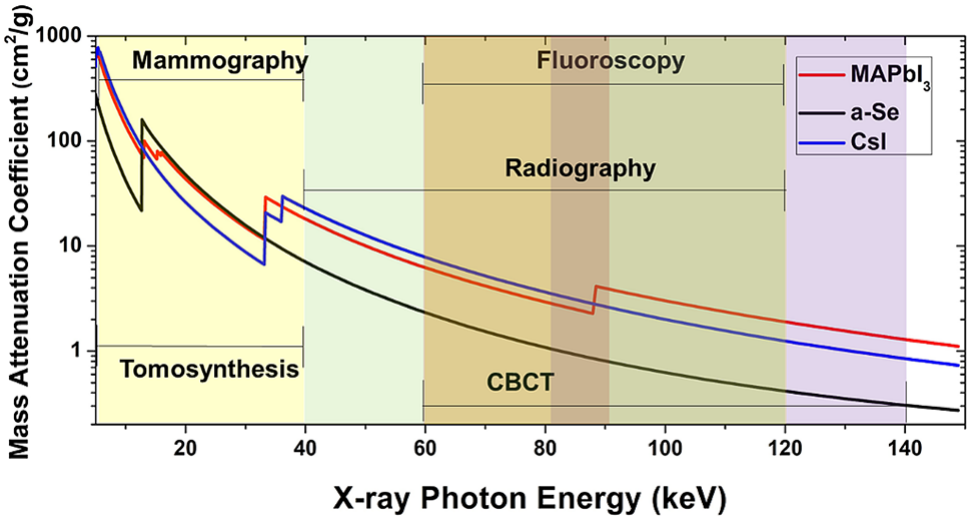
Mass attenuation coefficient versus X-ray energy for direct and indirect FPXI sensors, and MAPbI_3_ (calculations performed using NIST database). CBCT refers to cone beam CT.
